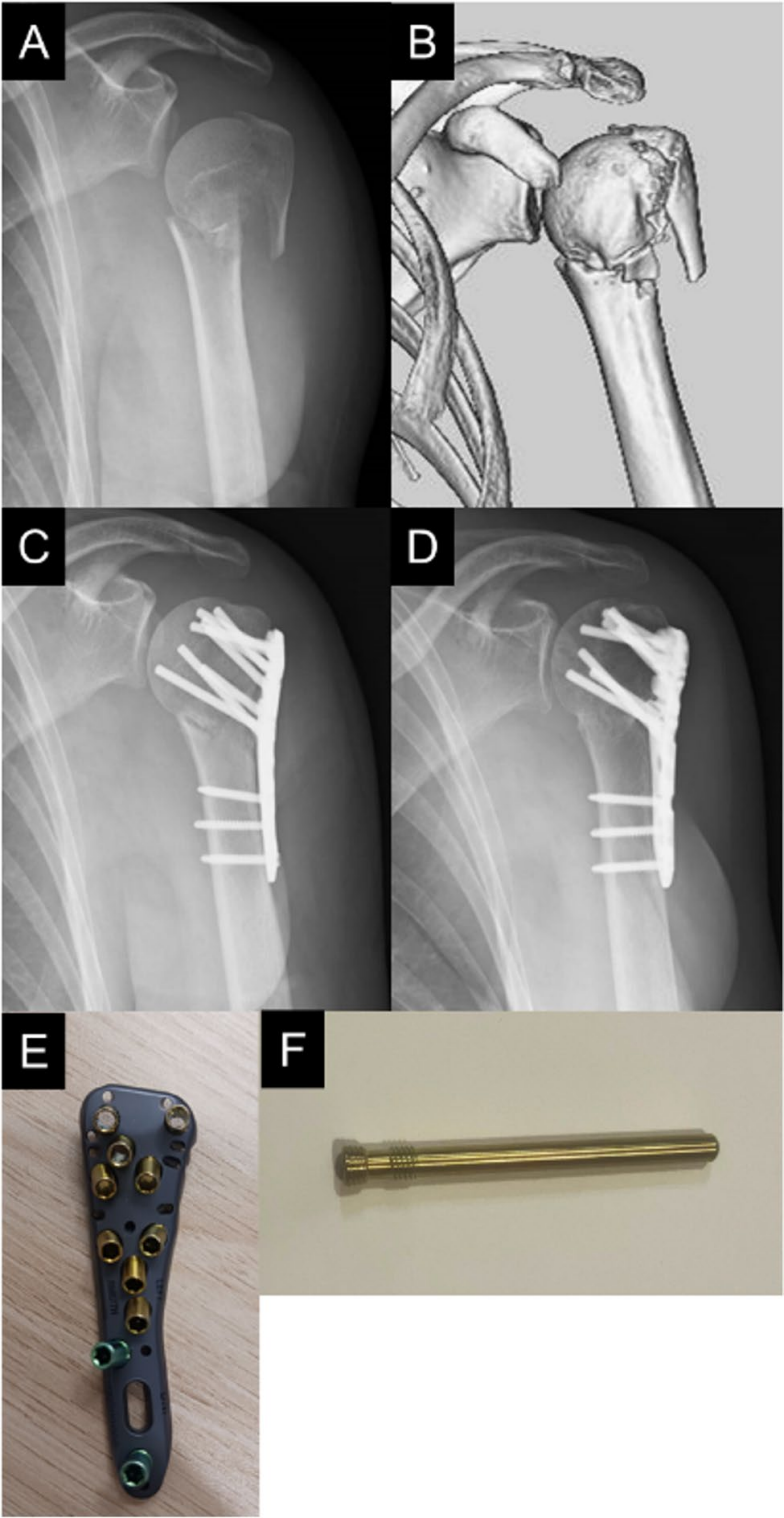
Clinical images and photographs of the plate fixation using ALPS plate with all screws. Preoperative radiograph (A), three-dimentional compuded tomography image (B), and radiograph immediately after surgery (C) and 1 year at postoperatively (D) of the patient who underwent plate fixation using ALPS plate with all screws. Photograph of ALPS plate (E) and proximal screw (F)
